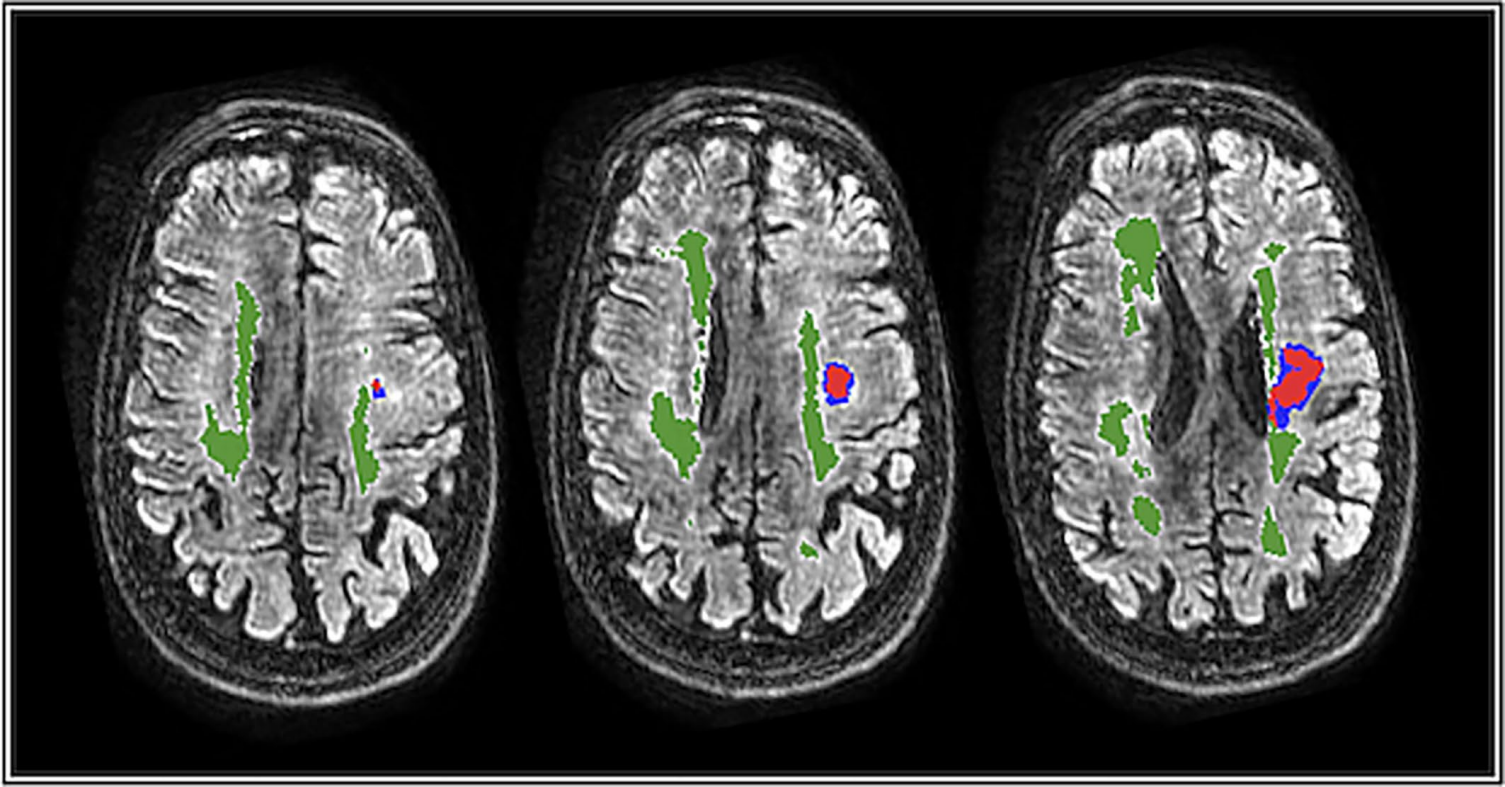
Example of WMH segmentation in green in a single patient across different horizontal cuts. Stroke lesion mask from DWI indicated in blue and red shows successful differentiation between WMH and stroke lesion.
